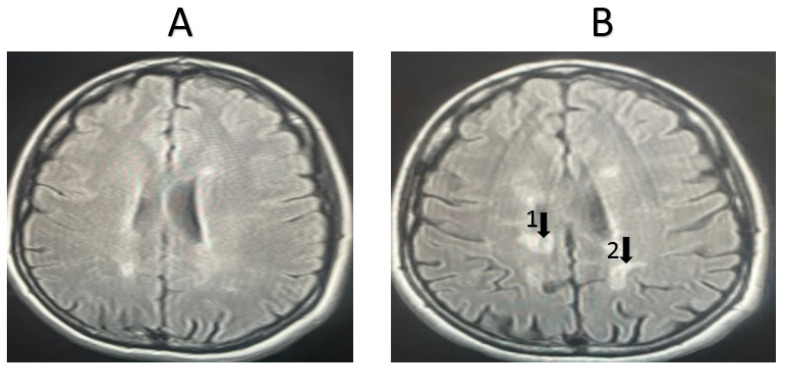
MRI examination of an MSO patient taking another medication (not fingolimod). (A). The number of lesions before treatment = 14, with an average lesion size (cm) of 0.35 ± 0.17, (B). The number of lesions after treatment = 18, with an average lesion size (cm) of 0.40 ± 0.19. The progression of the disease worsened after one year of treatment. Arrows refer to new lesions formed.
